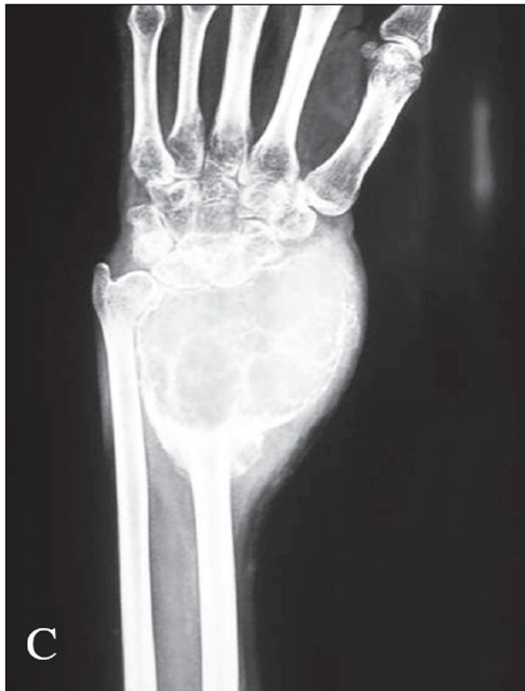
X-ray (antero-posterior) of wrist showing Campanacci grade 3 lesion: Deformed contour, cortical breach with soft tissue extension
